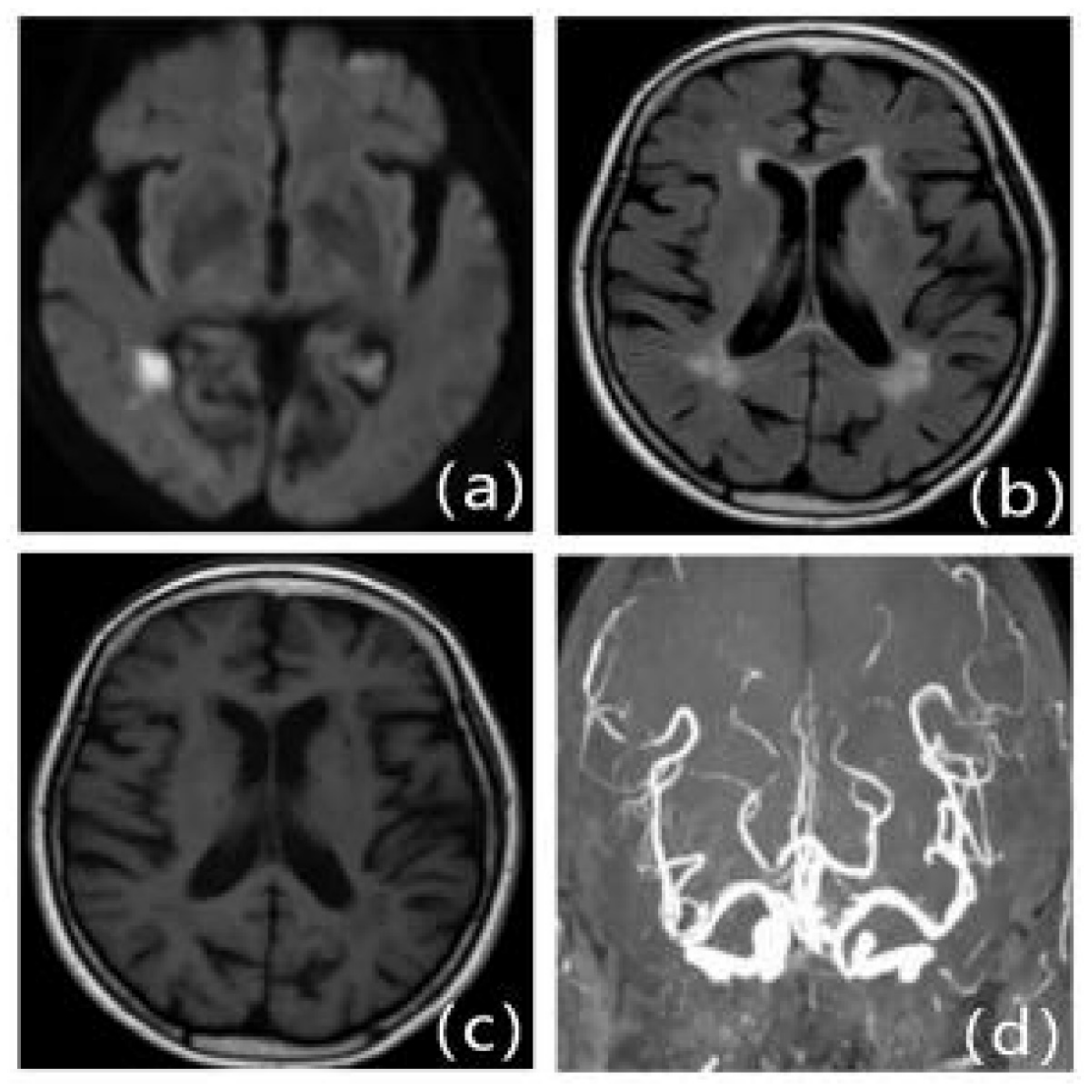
Patients with first IS due to ICAS with moderate LA: Female, 79 years old, admitted 6.5 hours after onset. A: DWI, B: T2WI-FLAIR, C: T1WI, D: MRA. DWI shows fresh cerebral infarction in the right temporo-occipital junction region; MRA shows multiple stenoses in the right posterior cerebrum. T2WI-FLAIR and T1WI show bilateral periventricular cerebral white matter demyelination changes.
